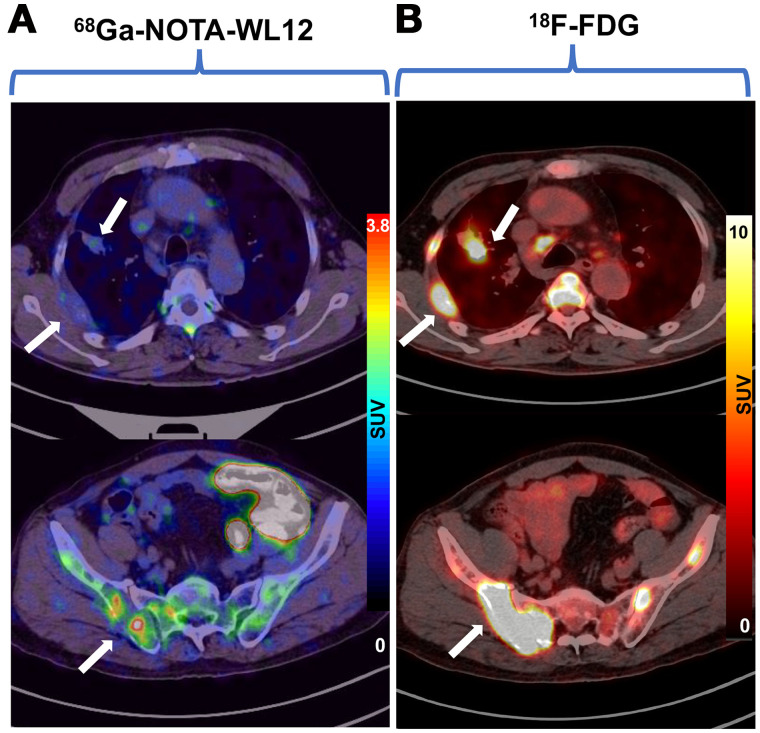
For patient 8, a 68-y-old man with lung adenocarcinoma, images showed inhomogeneous intra- and intertumoral uptake on ^68^Ga-NOTA-WL12 PET/CT (A) and high homogeneous uptake in tumors (white arrow) on ^18^F-FDG PET/CT (B).
